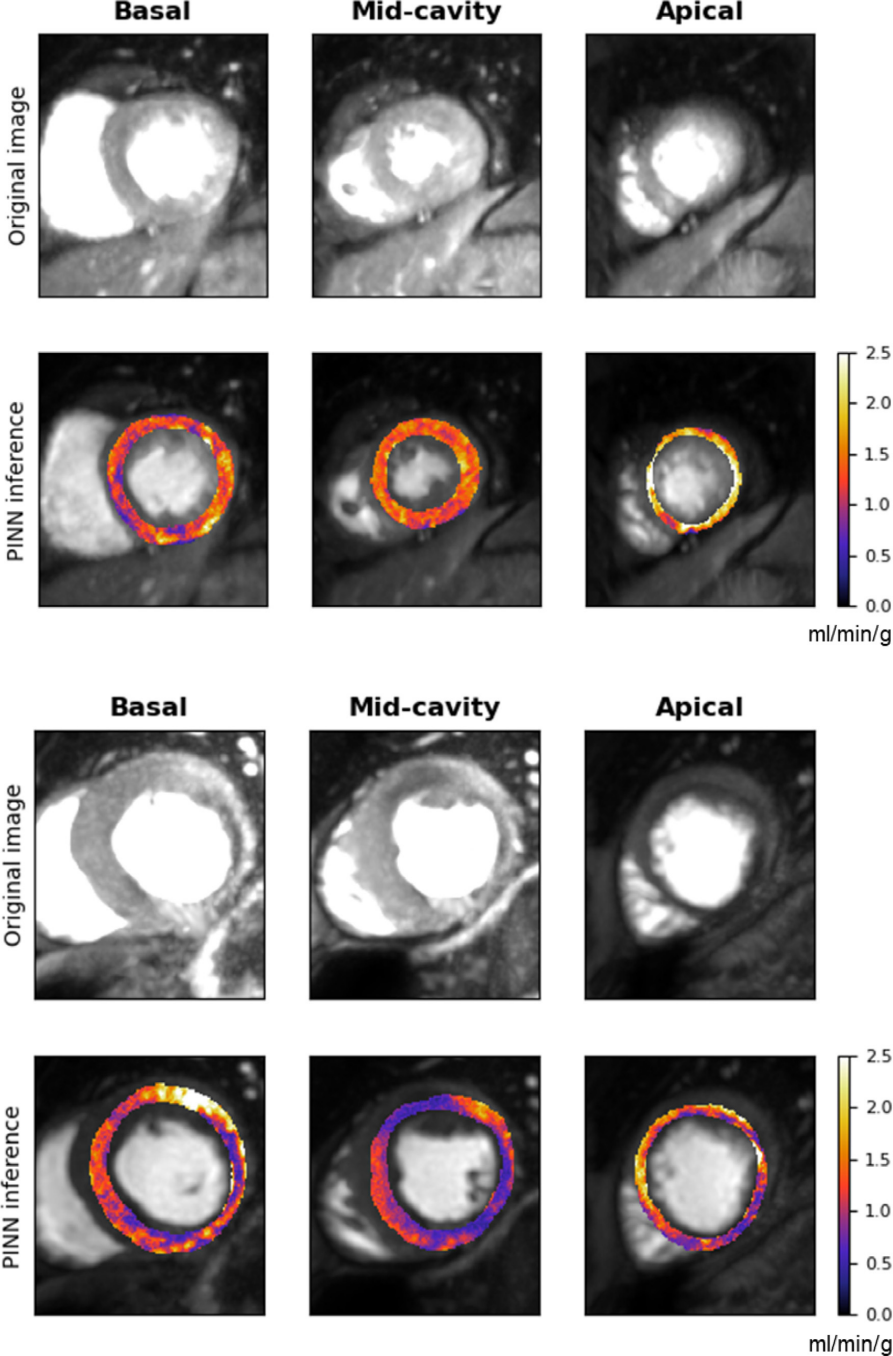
The estimated MBF maps for a patient (number 8) with no significant CAD (top) and patient (number 5) with CAD (bottom). The maps are shown under the corresponding MR images. The contrast of the MR images has been stretched to try to more clearly visualise the ischaemic regions.
